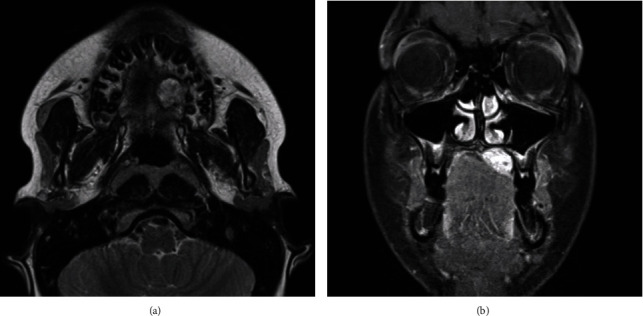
MRI sections showing a well-defined, homogenous enhancing lesion in the left palate.
